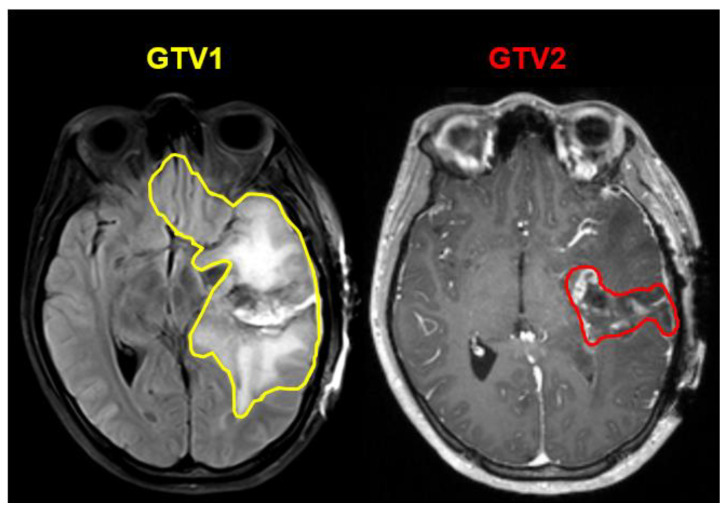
Example contour overlays from our training dataset of GTV1 (yellow contour) overlaid on T2w/FLAIR MRI and GTV2 (red contour) on CE-T1w MRI that includes cavity and blood product.
